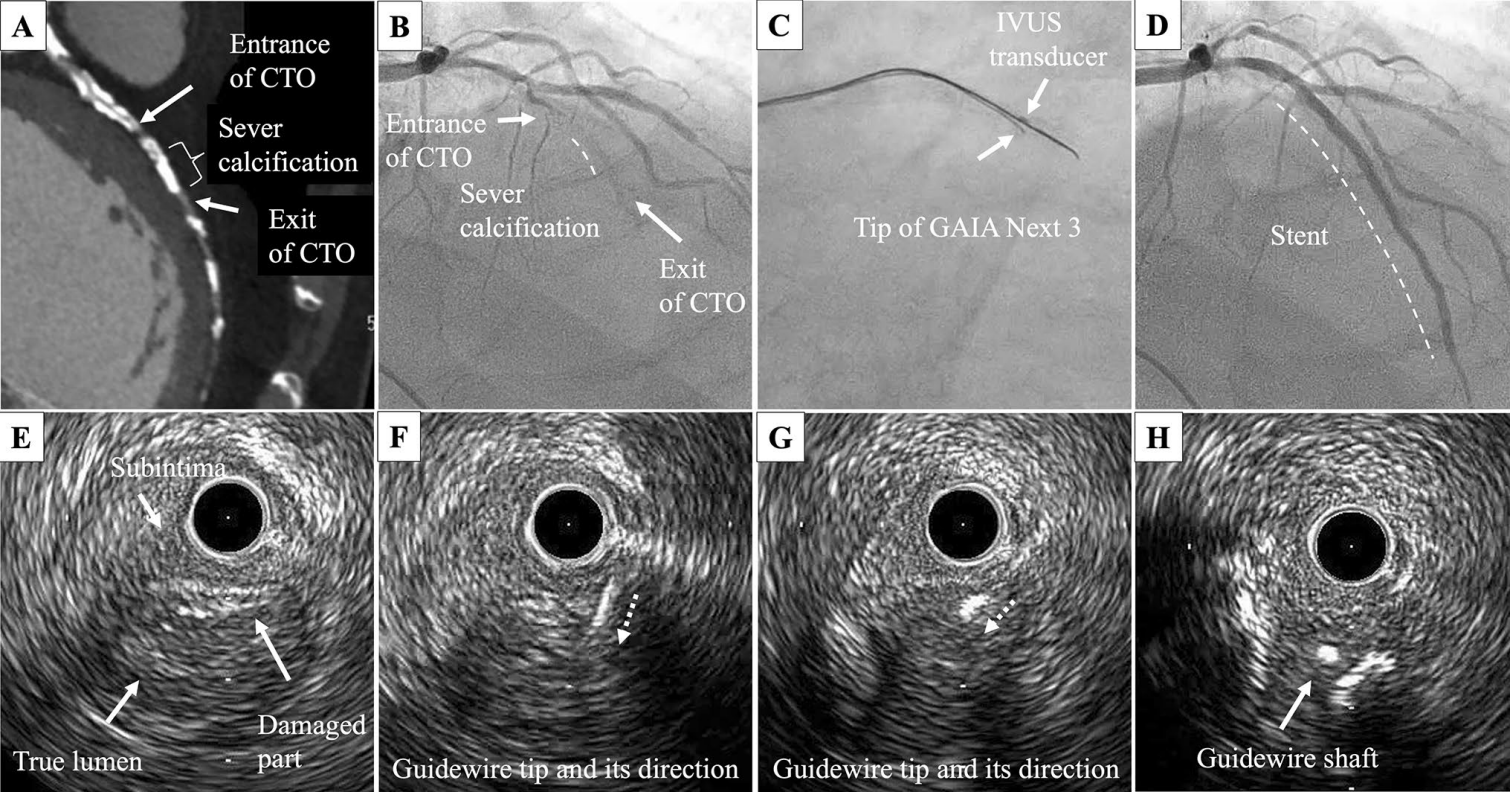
Computed tomographic image (A), angiographic images (B) prior to, C during, and D after the procedure, and intravascular ultrasound images E prior to, F, G during the puncture, and H after successful puncture. *CTO* chronic total occlusion
